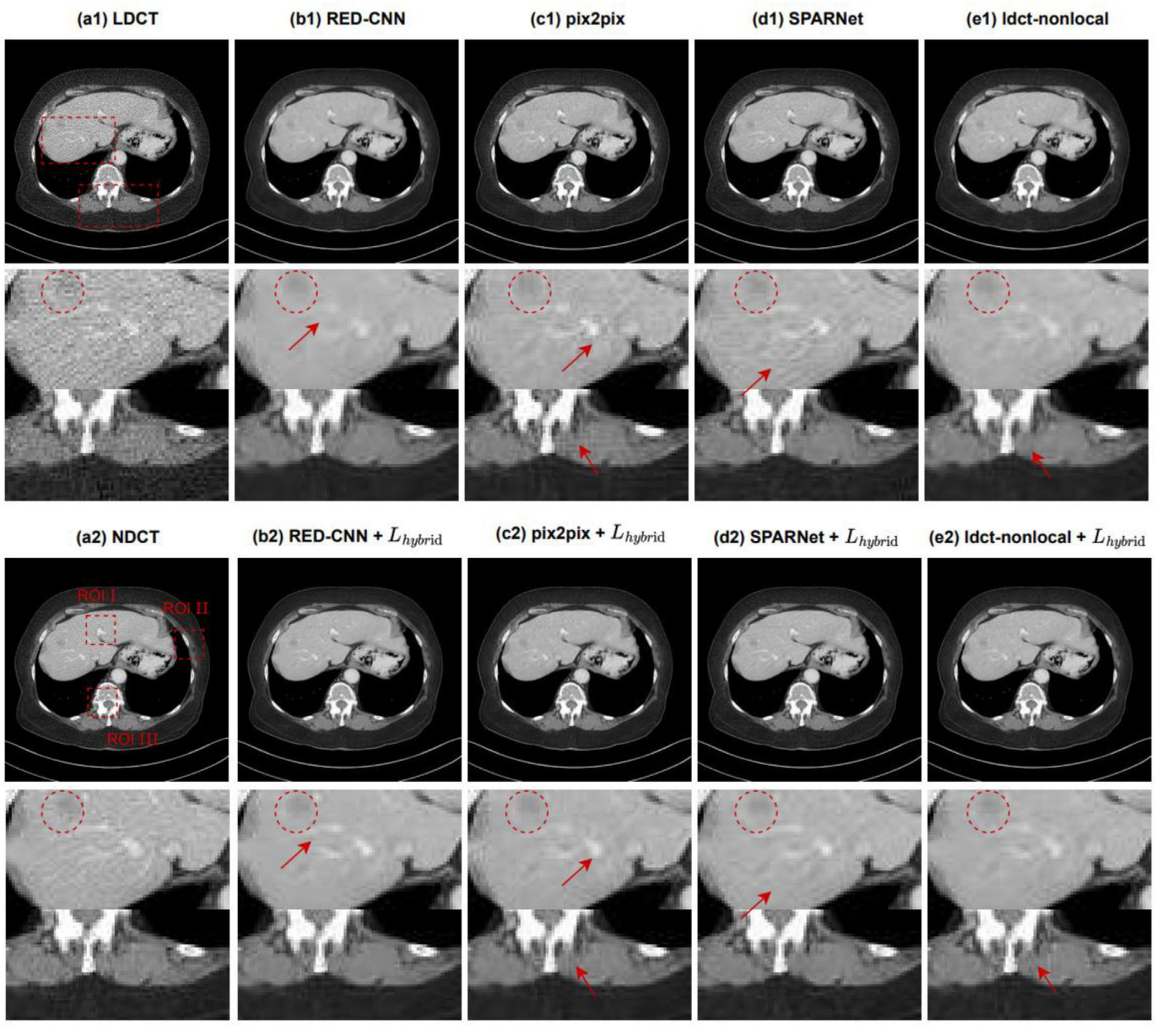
Impact of the hybrid loss function on each model for the abdominal slice in the testing dataset. The images from (a1) to (e1) are LDCT, and the denoising results of RED‐CNN, pix2pix, SPARNet, and ldct‐nonlocal before adding the hybrid loss function, respectively. The two red rectangles in LDCT specify the regions of interest (ROI) and the amplification results are shown in the second row. The images from (a2) to (e2) are the NDCT and the denoising results of each model after adding hybrid loss respectively, and the fourth row is the enlarged images of the corresponding ROI. The arrows indicate the areas where the visual effect are observed. The CT display window is [−160 240] HU. In contrast, after adding the hybrid loss function, every model retains more details and reduces artifacts and blur.
